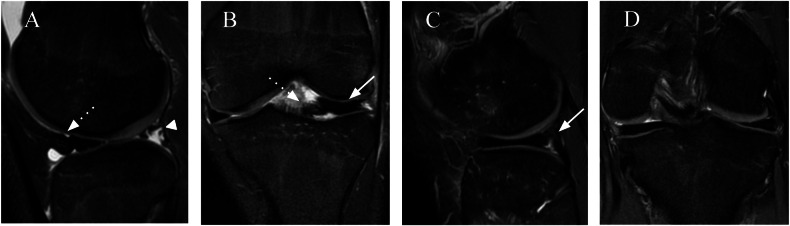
A 17-year-old male soccer player with a history of knee trauma and postsurgical suture evaluation 6 months later. A, B Sagittal and coronal T2FS: presurgical MR image showing detachment of the posterior horn of the meniscocapsular junction (arrowhead), anterior displacement of a portion of the meniscus (dotted arrow), and thickening of the anterior horn (arrow). C, D Sagittal and coronal T2FS: image 6 months after meniscal surgical suture showing posterior meniscocapsular repair (arrow) with good evolution and an appearance similar to that of the contralateral meniscus shown in D
